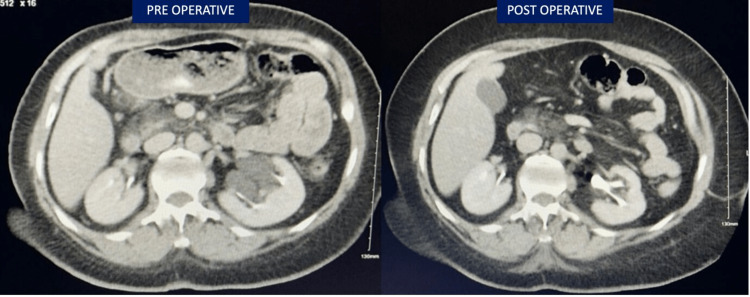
Follow-up CT scan after one year, compared with the pre-operative imaging.
